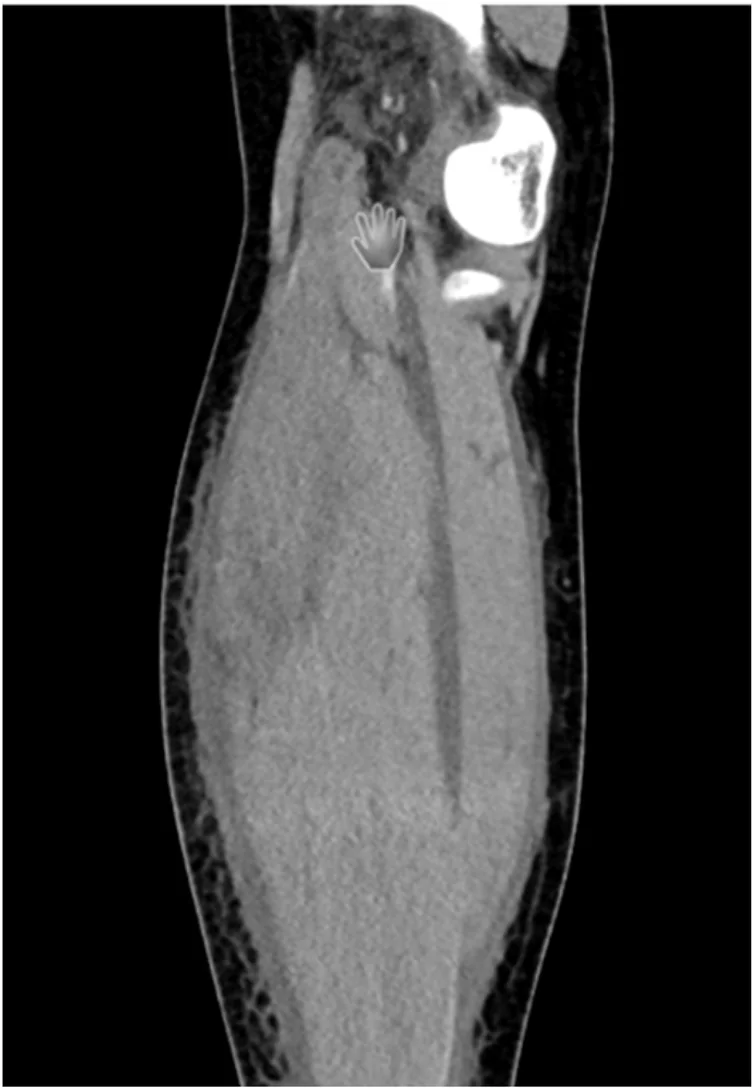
Coronal CT reconstruction in venous phase of the right calf, demonstrating perifascial fluid and hypodense areas within the right gastrocnemius muscle.
